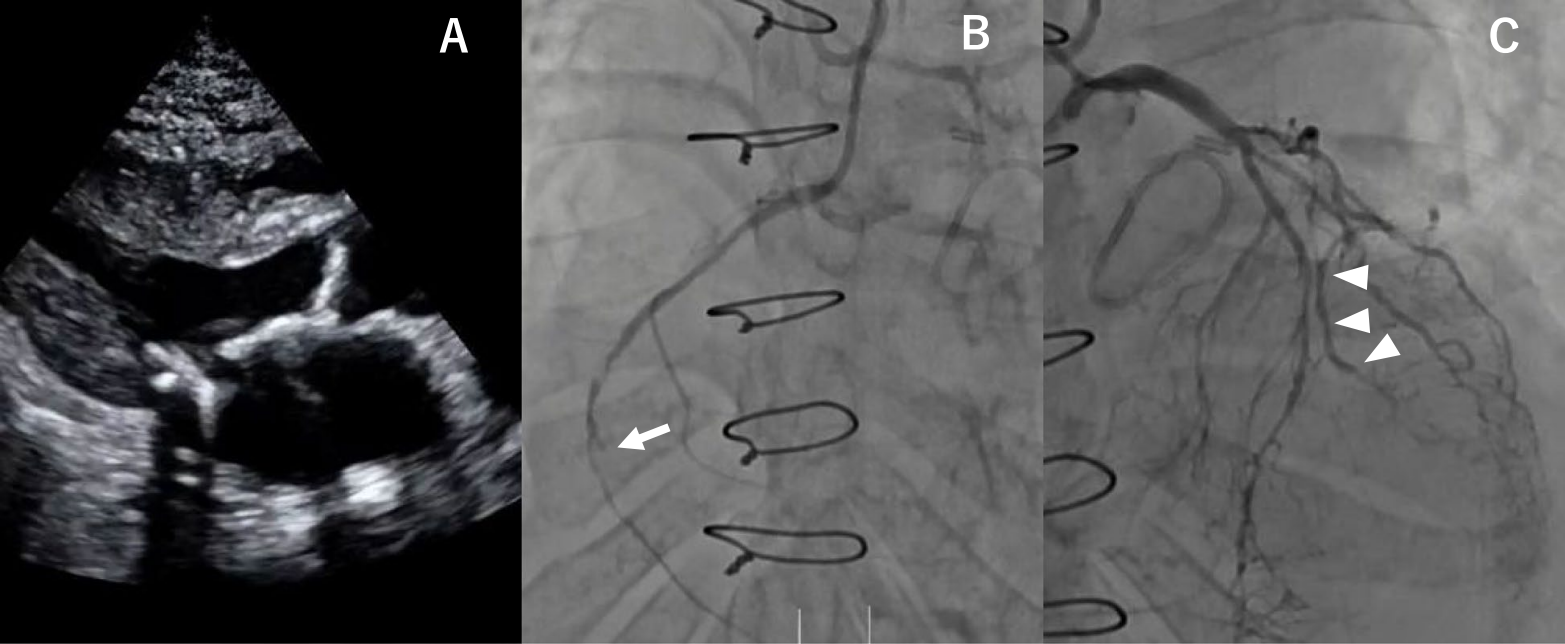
A Echocardiography revealed calcification with restricted mobility of both the aortic and mitral valves. B Coronary angiography showed occlusion of the right coronary artery (white arrow) and C the previous great saphenous vein graft(white arrow head)
